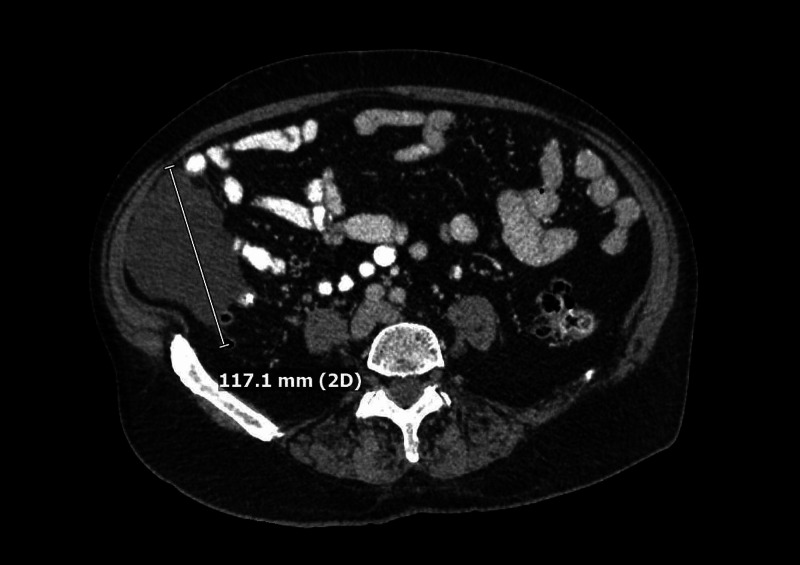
Abdominal CT (August 2019). CT of the abdomen showing a right paracolic gutter lobular fluid-attenuated structure with features of a cystic lymphangioma measuring 11.7 cm.
